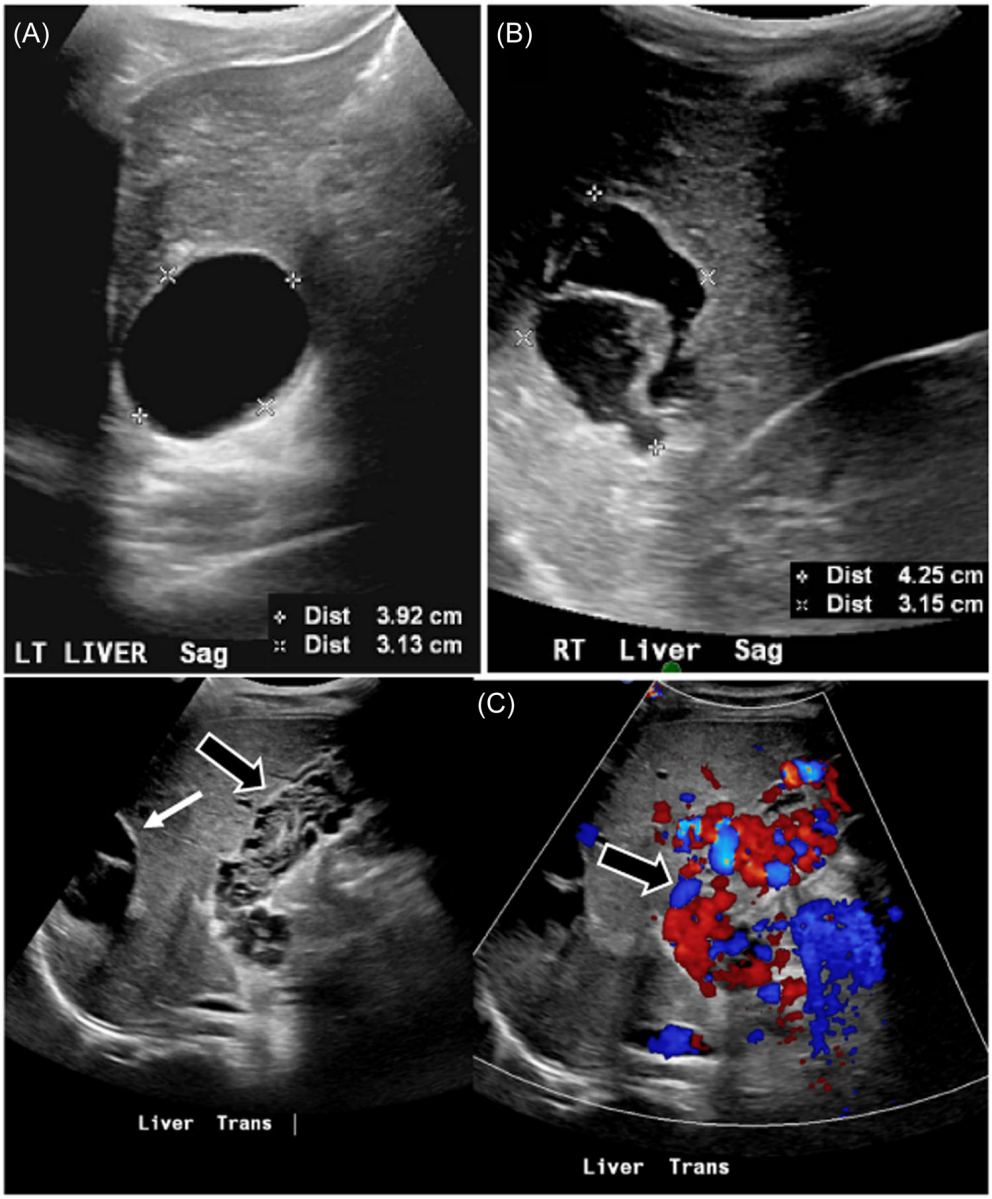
(A) Liver US image that revealed a unilocular, anechoic cystic lesion in segment II measuring 3.9 × 3.1 cm. (B) Liver US image that revealed “water lily” sign in cystic lesion in segment VI/VII measuring 4.2 × 3.1 cm. (C) Gray scale and color doppler US images show striking varices within gallbladder wall (thick arrows) as well as a portion of the cystic lesion in the right liver (thin arrow).
